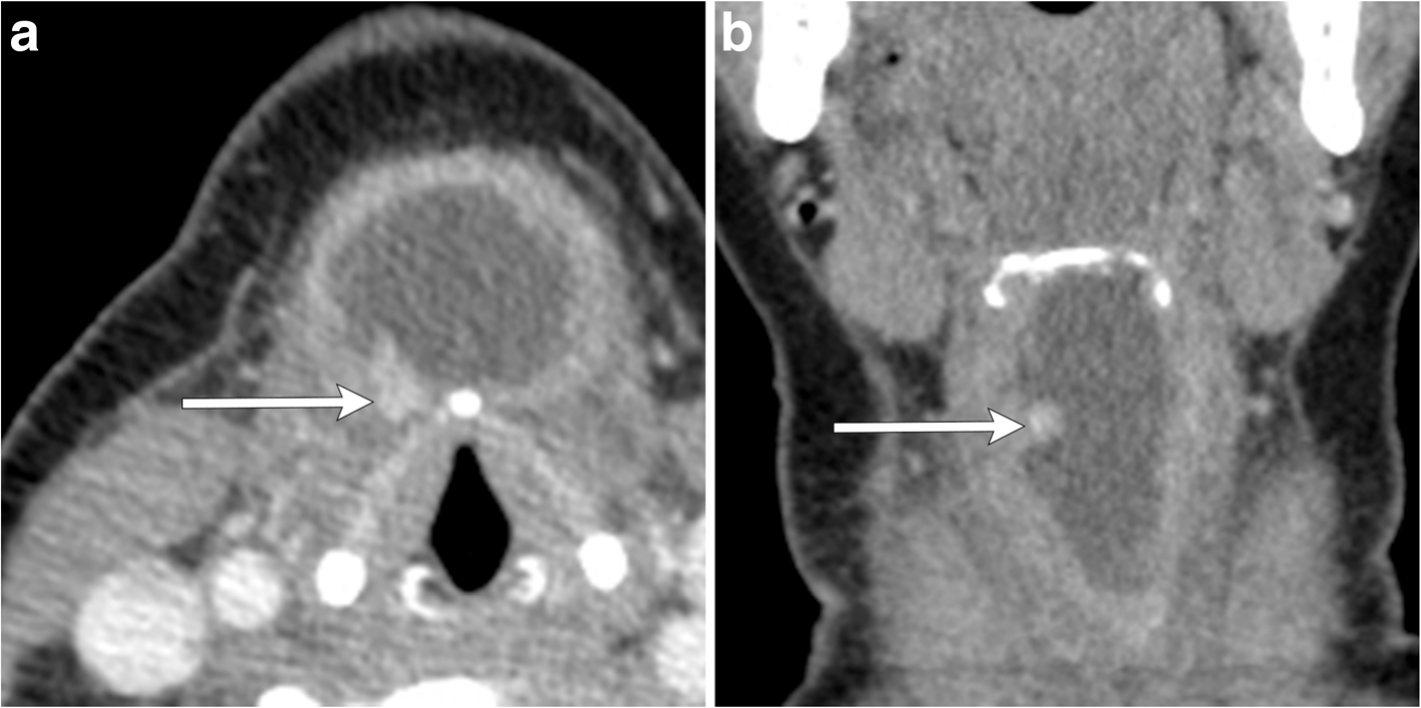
Thyroglossal ductal cyst with malignancy. Axial (a) and coronal (b) contrast-enhanced CT images of the neck show an irregularly thick-walled thyroglossal duct cyst with enhancing mural nodule (arrows). Resection revealed papillary carcinoma
